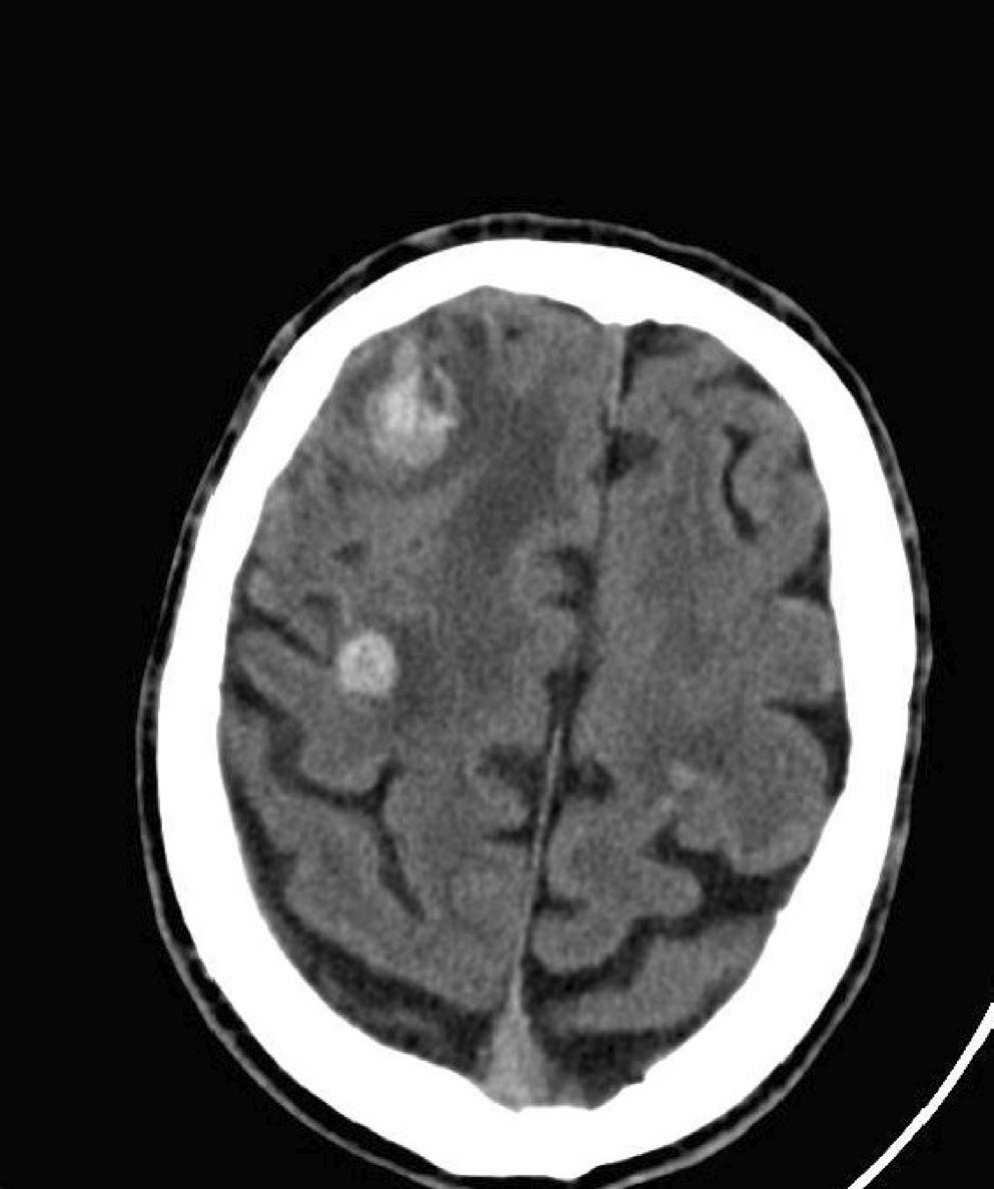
FIGURE 3 CASE 2 Noncontrast enhanced computed tomography revealing several intraparenchymal hyperdense rounded lesions and surrounding vasogenic edema.
